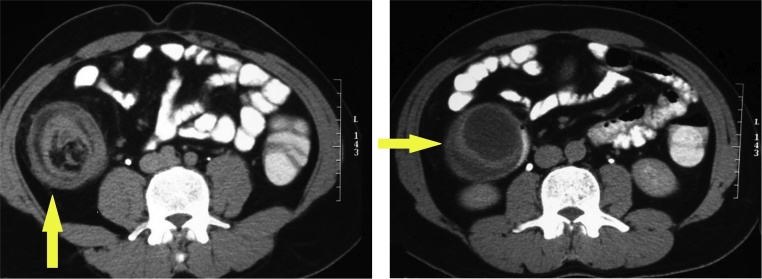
Contrast-enhanced abdominal computed tomography CT showing a cystic lesion within the lumen of cecum with thick septations (yellow arrow) (For interpretation of the references to colour in this figure legend, the reader is referred to the web version of this article).
